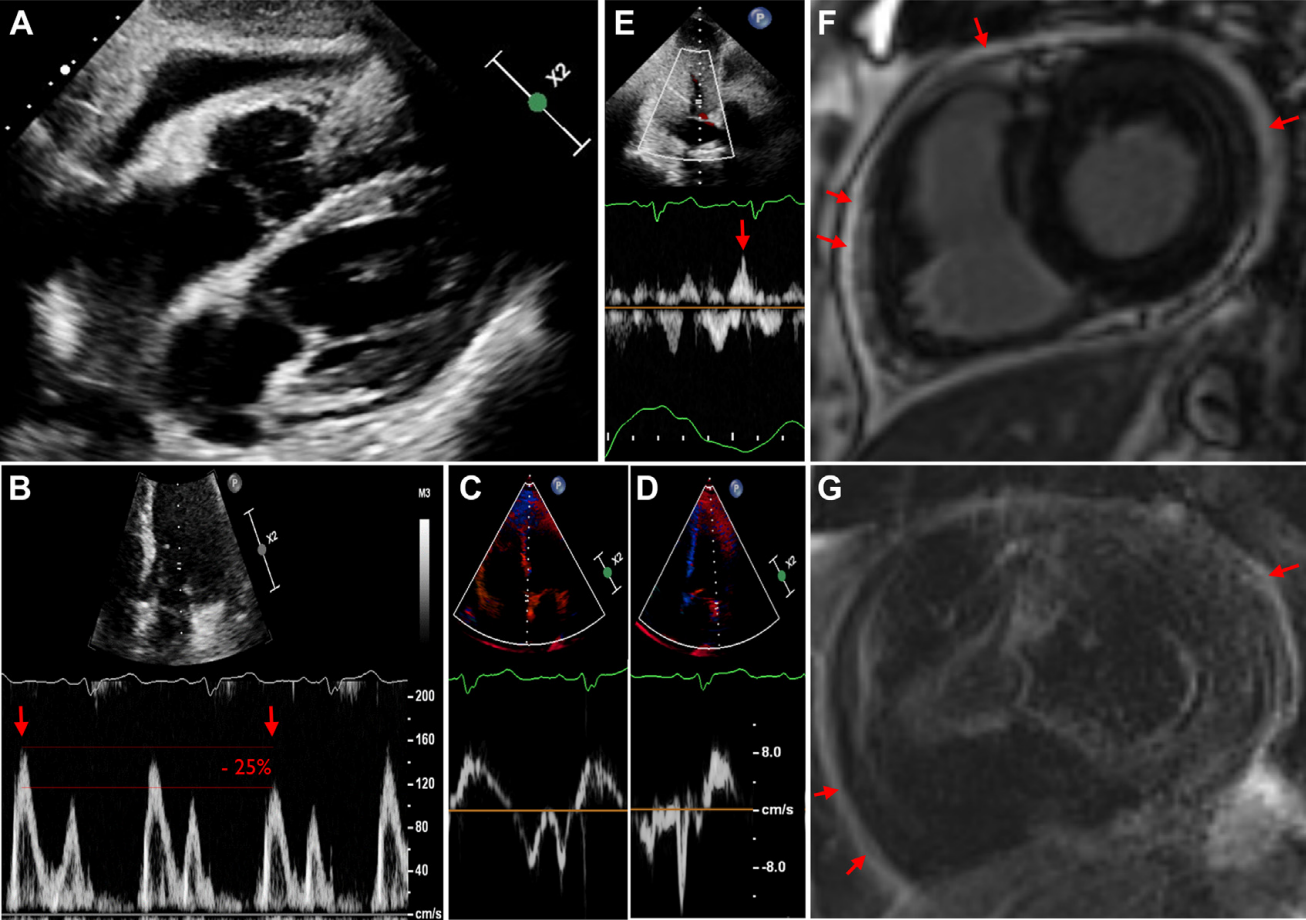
Imaging Findings of Patient 2 (A) Transthoracic echocardiography subcostal views show moderate circumferential pericardial effusion without diastolic compression of right heart chambers. (B) Pulsed wave Doppler demonstrates significant respiratory variation in mitral early diastolic inflow velocity (25% decrease with inspiration, red arrows). (C) Septal mitral annular tissue Doppler velocity (e'_med_ = 8 cm/s) exceeding the (D) lateral mitral annular tissue Doppler velocity (e'_lat_ = 6 cm/s), demonstrating annulus reversus. (E) Pulsed wave Doppler sampling of the hepatic veins demonstrating end-diastolic expiratory flow reversal (red arrow). (F) Cardiac magnetic resonance images 15 days after admission display pericardial thickening and delayed gadolinium enhancement in phase sensitive inversion recovery sequences (red arrows). (G) T2 black blood sequences demonstrate pericardial edema (red arrows).
